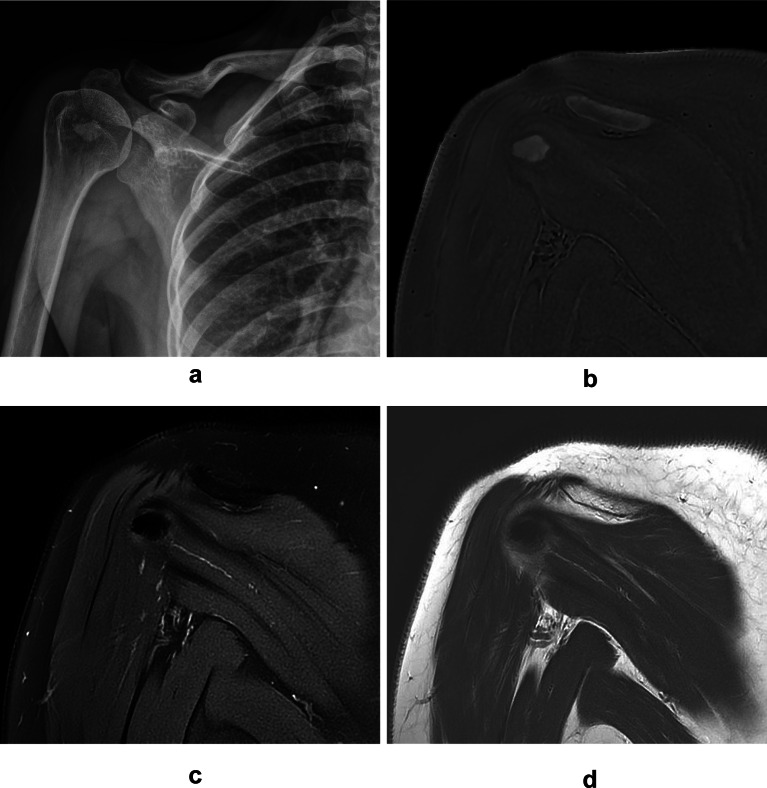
A 40-year-old patient with calcific tendinitis in formative phase at infraspinatus tendon of shoulder. Radiography (A) showed a well-defined, homogeneous type 2 calcific deposits by Depalma’s classification. Coronal ZTE image (B) showed a oval shaped, well-defined calcific deposit with homogeneous high signal intensity. Coronal FSPDWI (C) showed a oval shaped, well-defined calcific deposit with low signal intensity. Coronal T2WI (D) showed a smaller sized calcific deposit with indistinct margin and similar low signal intensity with surrounding infraspinatus tendon.
